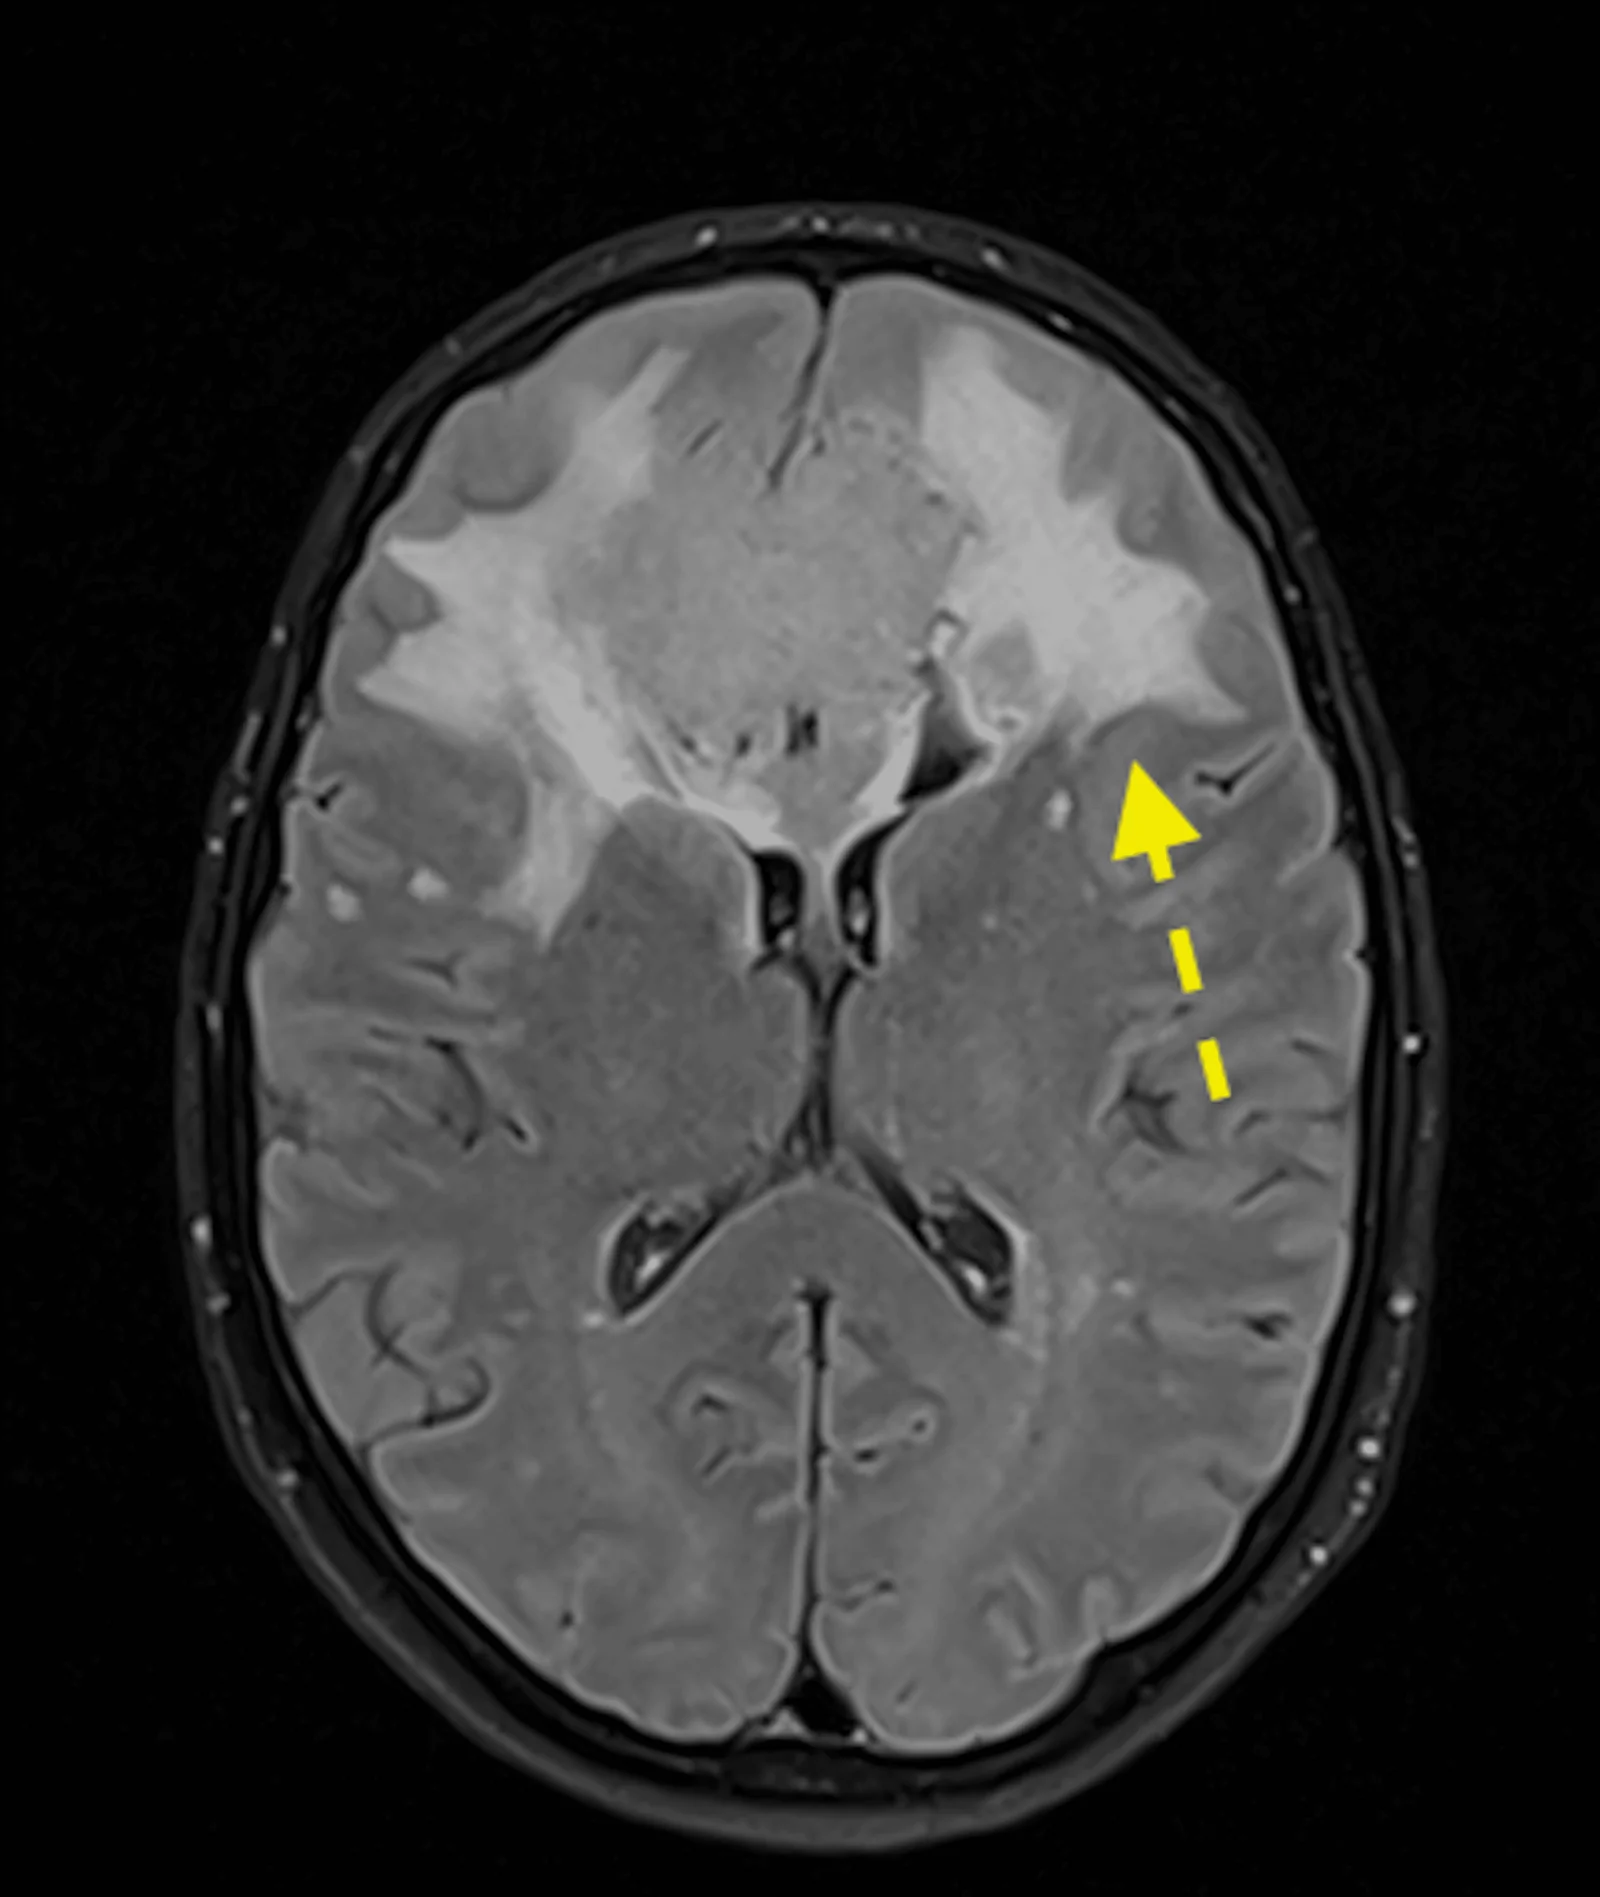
Preoperative axial T2-weighted FLAIR brain MRI Extensive bilateral frontal vasogenic edema, greater on the left, is seen adjacent to the anterior cranial fossa mass, with associated sulcal effacement and local mass effect. The dashed arrow indicates the peritumoral edema FLAIR: fluid-attenuated inversion recovery; MRI: magnetic resonance imaging
